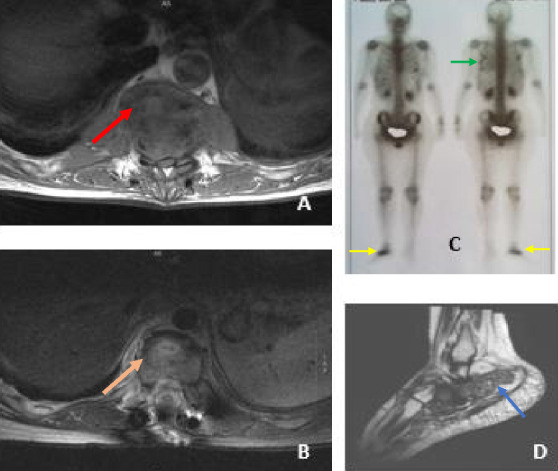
(A, B) MRI of Case 8 showing thoracic tuberculosis before (red arrow) and 12 months after treatment initiation-improvement (orange arrow). (C) Gallium scan of Case 3 showing TB of the ribs (green arrow) and right ankle joint (yellow arrows). (D) MRI of Case 3 showing ankle joint TB involvement (blue arrow)—same case as in (C).
